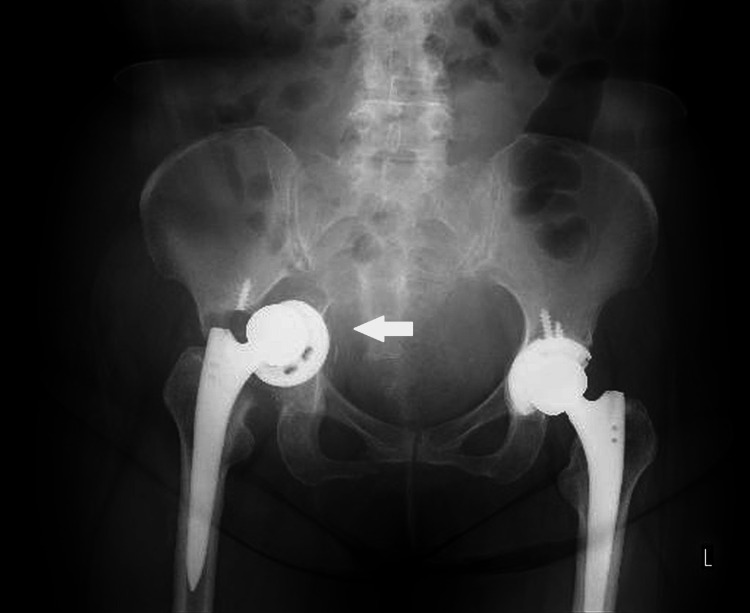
Plain radiograph of the pelvis after the secondary fall (anteroposterior view). Progressive displacement of the fracture was evident (arrow), with central dislocation of the femoral head and breakage of the acetabular cup screws.
